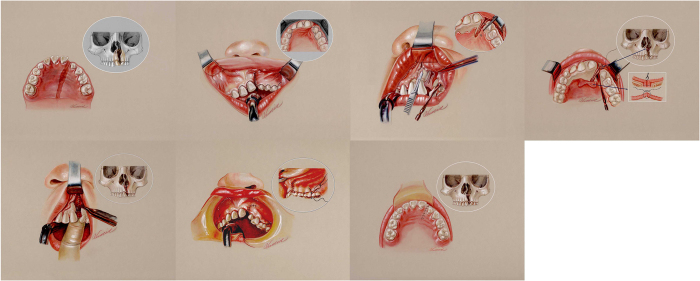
Left upper first and second: the alveolar bone defect was defined. The anatomic defect requiring alveolar bone grafting was also confirmed with three dimensional facial CT scans. Left upper third: A superiorly based gingival mucoperiosteal flap was raised to explore the alveolar bone defect. Left upper fourth: the palatal mucoperiosteal flap was raised to a level beyond the deepest margin of the alveolar fistula. Left lower first: the nasal floor tissue was completely closed. The bone graft was packed into the defect area. Left lower second and third: complete closure of gingival and palatal wounds.
